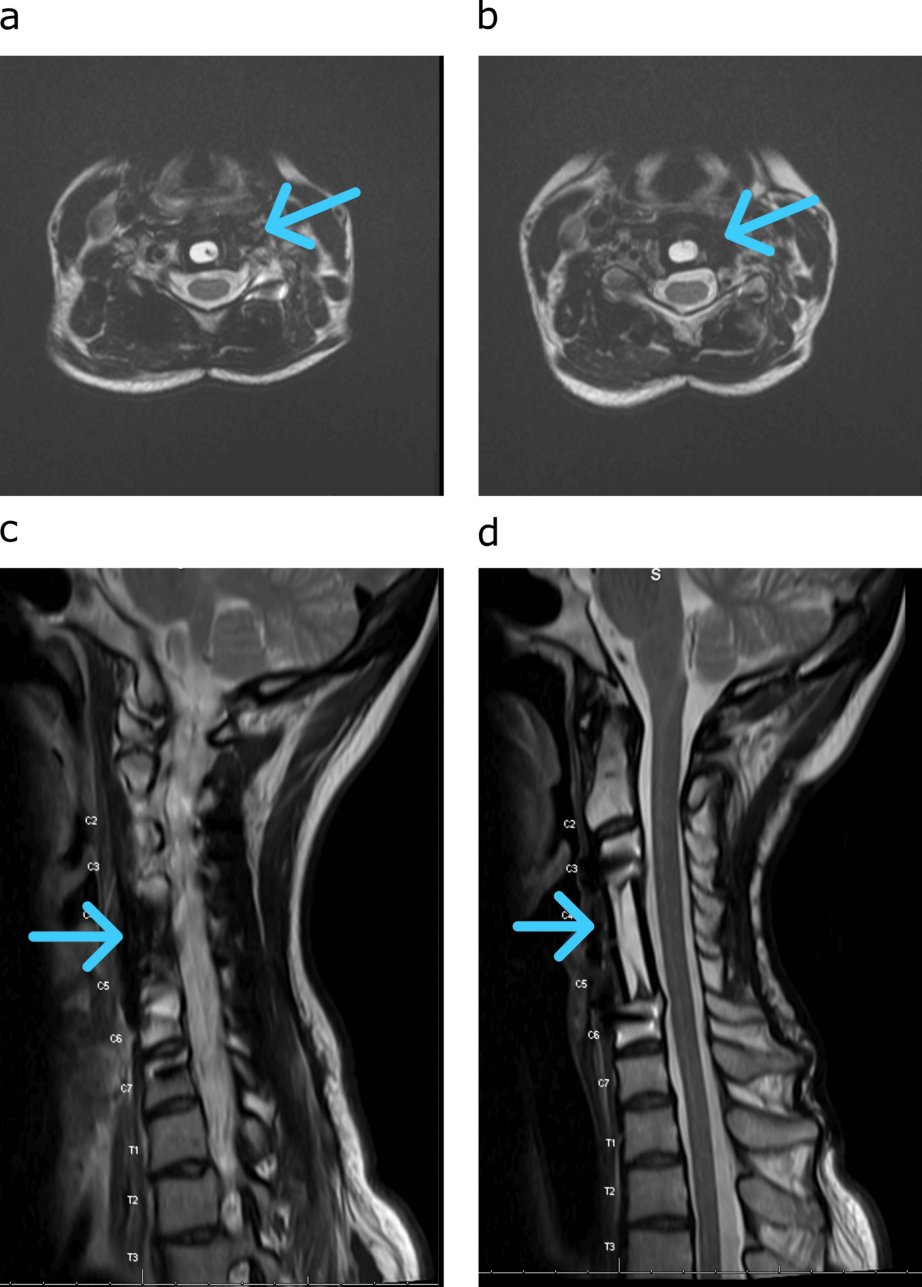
Post-procedure follow-up MRI scans Delayed 27-month follow-up MRI studies demonstrating continued resection and local control of the cervical chordoma. (a and b) T2 contrast-enhanced axial images at two different slices (blue arrows with the absence of tumor). (c and d) T2 contrast-enhanced sagittal images at two different slices (blue arrows with the absence of tumor). MRI: magnetic resonance imaging
